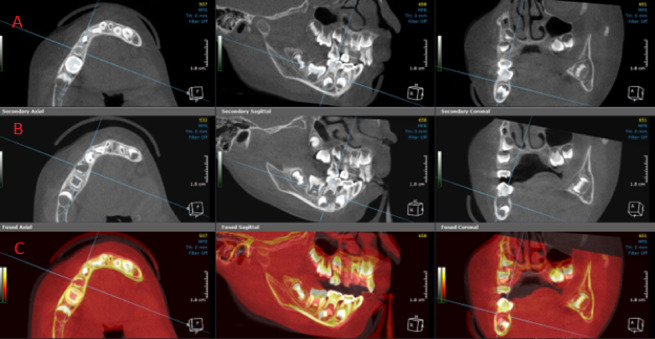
Fusion On Demand module.* (A) Cone-beam computed tomography (CBCT) image obtained immediately after restoration (baseline), (B) CBCT image at the 12-month follow-up, and (C) superimposed scans demonstrating parallel alignment of the baseline CBCT with the 12-month scan.
